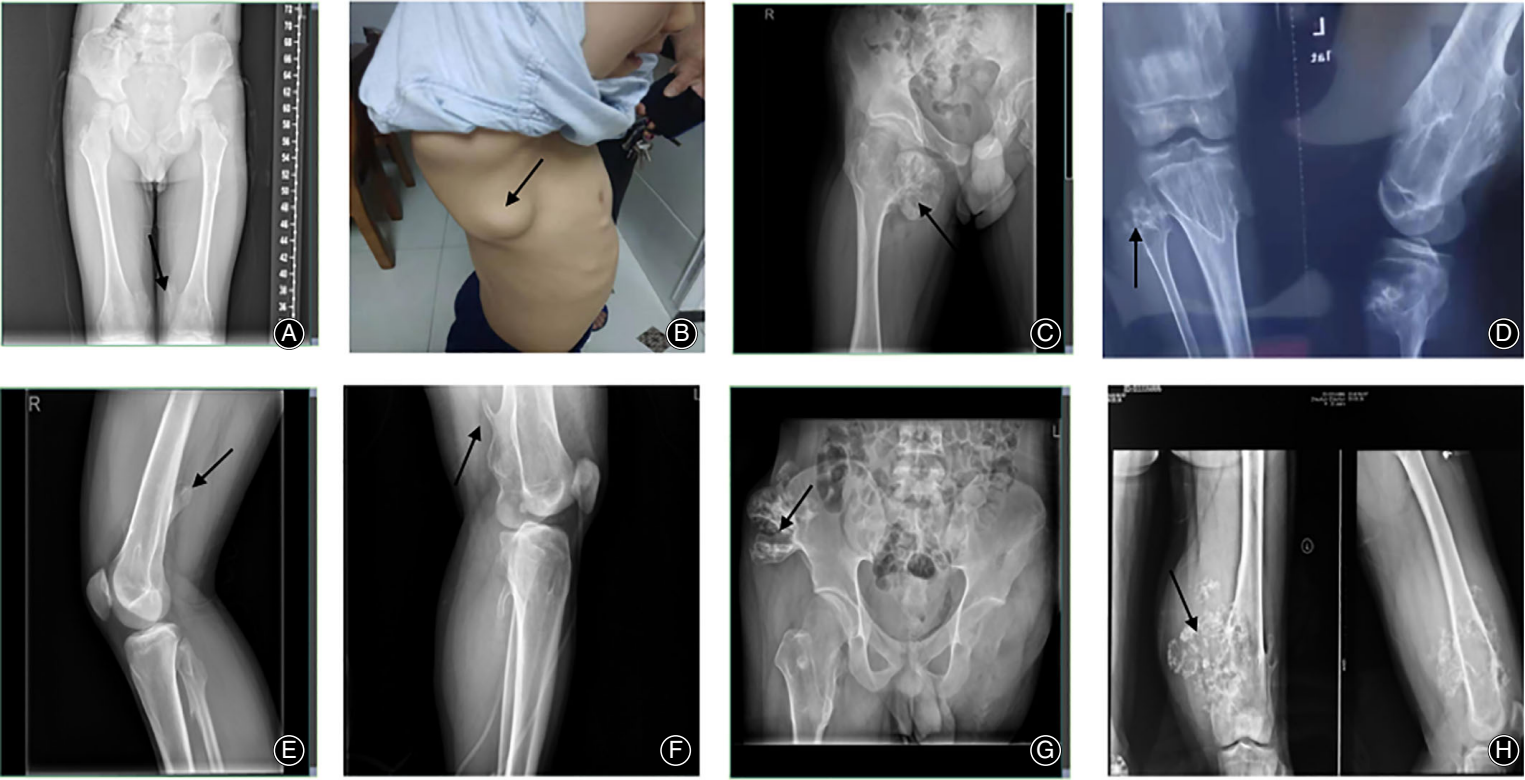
Appearance and radiology of the probands. (A) F1: X‐rays shows multiple exostoses on bilateral distal femur; (B) F2: One obvious bony prominence on his right rib; (C) F3: A huge exostosis on the proximal femur affecting the activation of hip joint; (D–F) (F4–F6, respectively): The X‐ray plain film shows multiple exostoses near the knee requiring surgery; (G) F7: a cauliflower‐like exostosis on the top of the pelvis; (H) F7: The plain radiograph of the distal femur showed extensive osteolytic lesions and obvious calcifications with a soft‐tissue mass.
